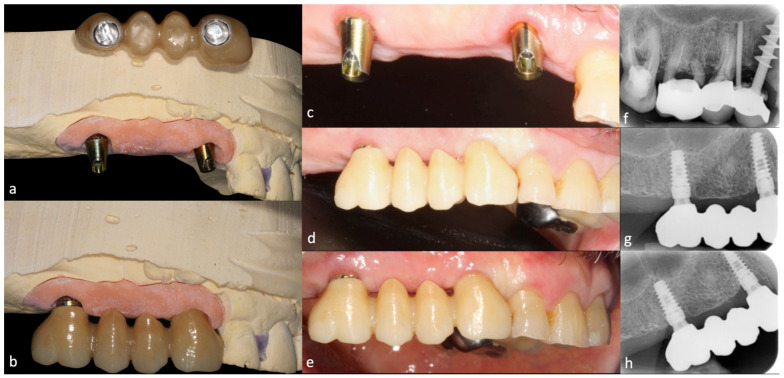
Cast models (a,b). Clinical images of a representative case at baseline (c,d) and after 12 months (e). Initial periapical radiographs of the initial situation (f), after six months (g), and after twelve months (h).
